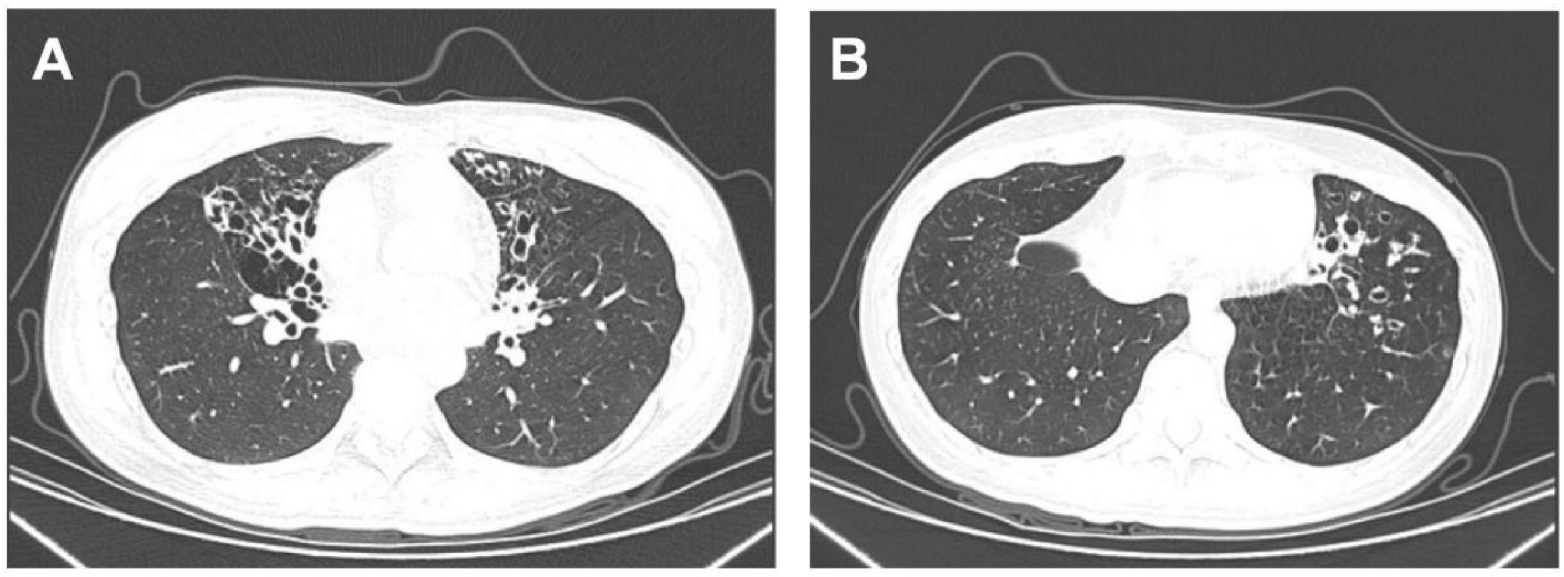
Chest CT of the proband. (A) Chest CT showed multiple cystic and cylindrical bronchiectasis with wall thickening in the right middle lobe and left lingular lobe. (B) Chest CT showed multiple cystic and cylindrical bronchiectasis with wall thickening in the left lower lobe.
